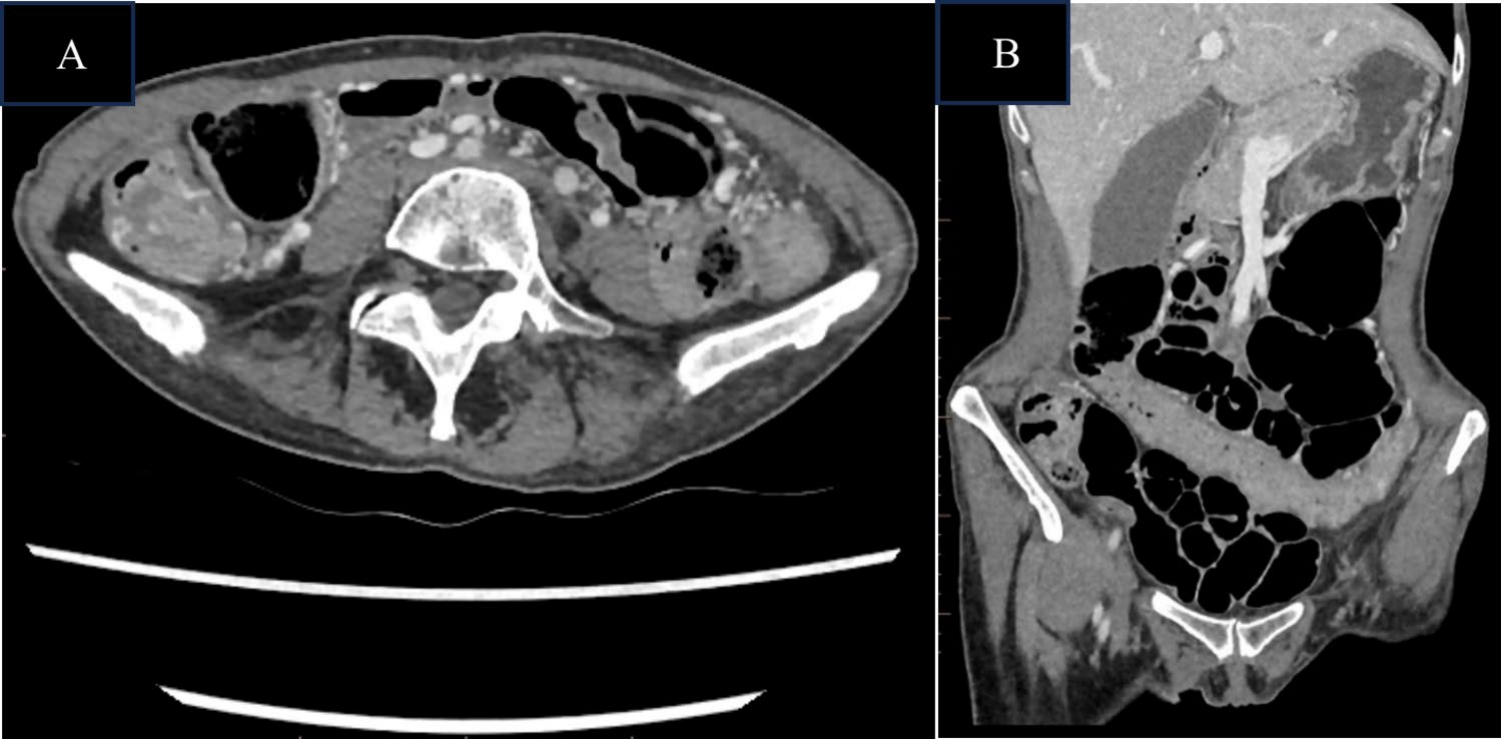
CT-abdomen and pelvis with intravenous contrast shows no gross abnormalities of the gastrointestinal tract within the limits of the study. A Axial plane. B Coronal plane
